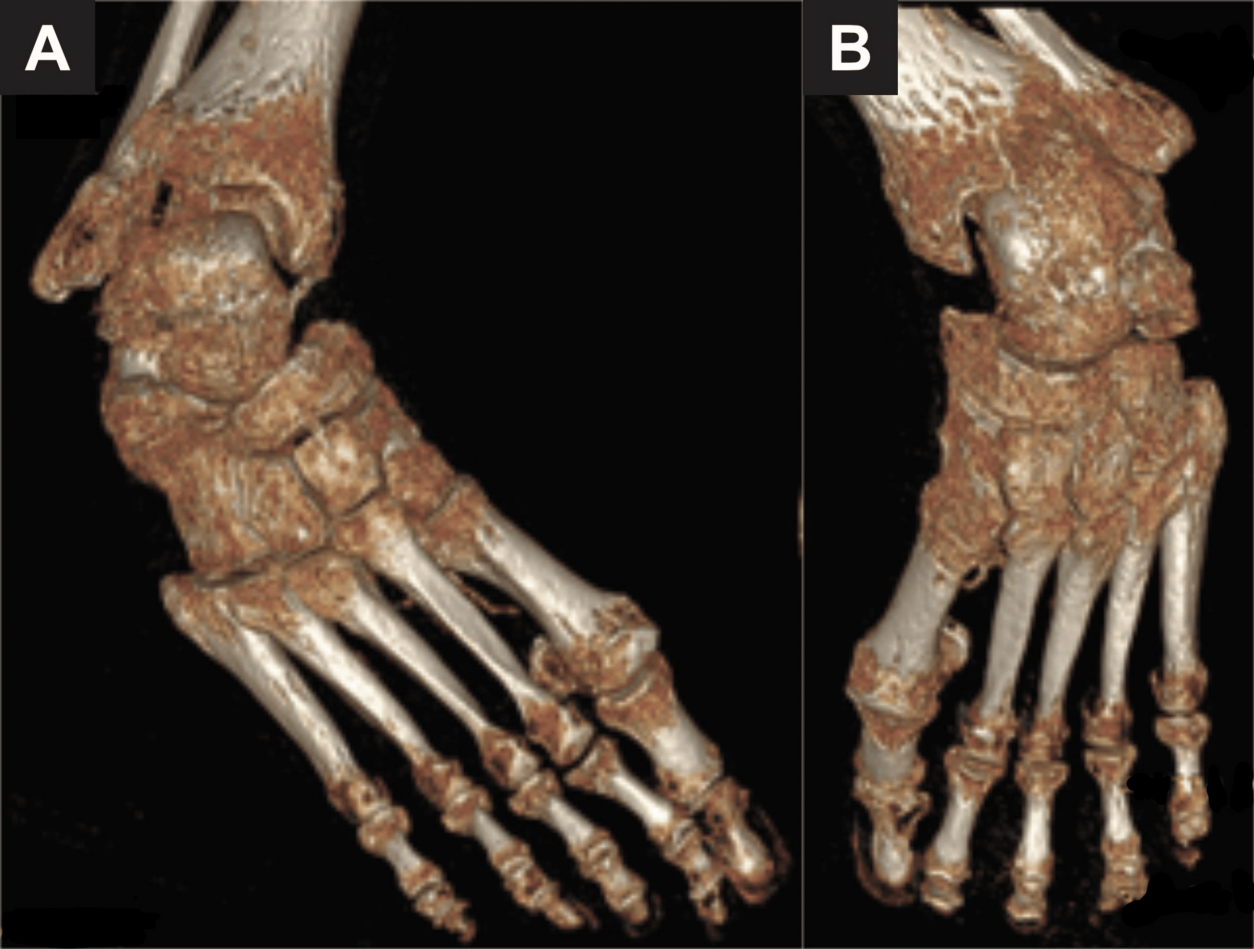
Preoperative CT of bilateral feet A: Right foot, B: left foot Three-dimensional reconstructed CT images demonstrating the right (A) and left (B) feet showing severe, rigid equinovarus deformities. Both sides reveal marked joint incongruity, midfoot collapse, and significant degenerative changes at the tibiotalar and subtalar joints. CT: computed tomography
